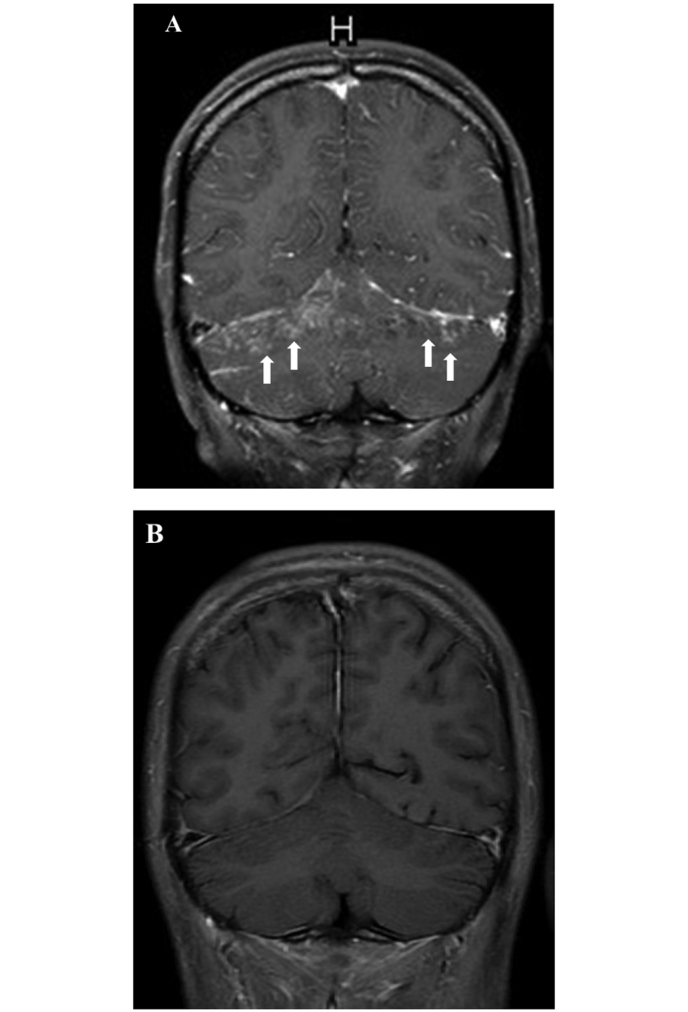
Gadolinium diethylenetriaminepentacetate-enhanced coronal T1-weighted image of the brain. (A) Diffuse leptomeningeal enhancement (white arrows) in the sulci of the bilateral cerebri and cerebelli. (B) Significant regression of the leptomeningeal enhancement, observed following treatment with dasatinib and intrathecal chemotherapy.
